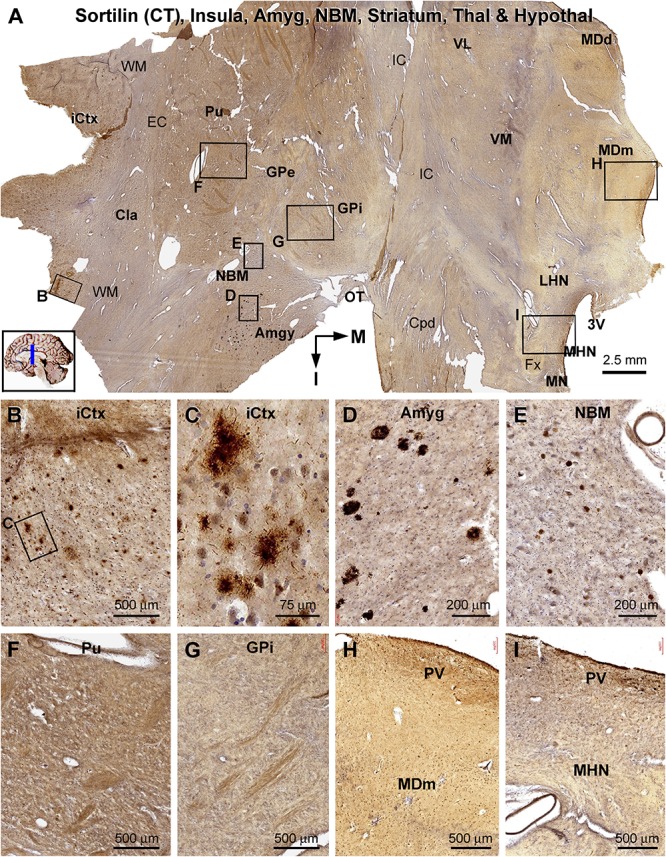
Lack of sorfra plaques in the striatum and diencephalon in an AD brain exhibited Thal phase 5 and Braak V stage neuropathologies. Section orientation, anatomical regions, figure panel arrangement, and scale bars are as indicated in individual panels. (A) A low-magnification image of the immunolabeled section (counterstained with toluidine blue) at the level of the mammillary nucleus (MN), displaying sortilin C-terminal (CT) antibody labeling in multiple telecephalic and diencephalic structures. Framed areas in (A) are enlarged and shown as (B–I). Sorfra plaques are present in the insular cortex (iCtx) (B,C) and the amygdaloid complex (Amyg) (D). Large neuronal somata are labeled in the nucleus basalis of Meynert (NBM), while no plaque profiles are seen in this basal forebrain nucleus (E). There are also no sorfra plaques present in the claustrum (Cla), and all the striatal, thalamic, and hypothalamic subregions, such as the putamen (Pu) (F), the external (GPe) and internal (GPi) divisions of the globus pallidus (GP) (A,G), the dorsal (MDd) and medial (MDm) divisions of the mediodorsal nucleus (MD) of thalamus (A,H), and the lateral (LHN) and medial (MHN) hypothalamic nucleus (HN) (A,I). Abbreviations: EC, external capsule; Fx, fornix; IC, internal capsule; VL, ventrolateral thalamic nucleus; VM, ventromedial thalamic nucleus; Cpd, cerebral peduncle; OT, optic tract; 3V, third ventricle; PV, paraventricular nucleus; WM, white matter.
